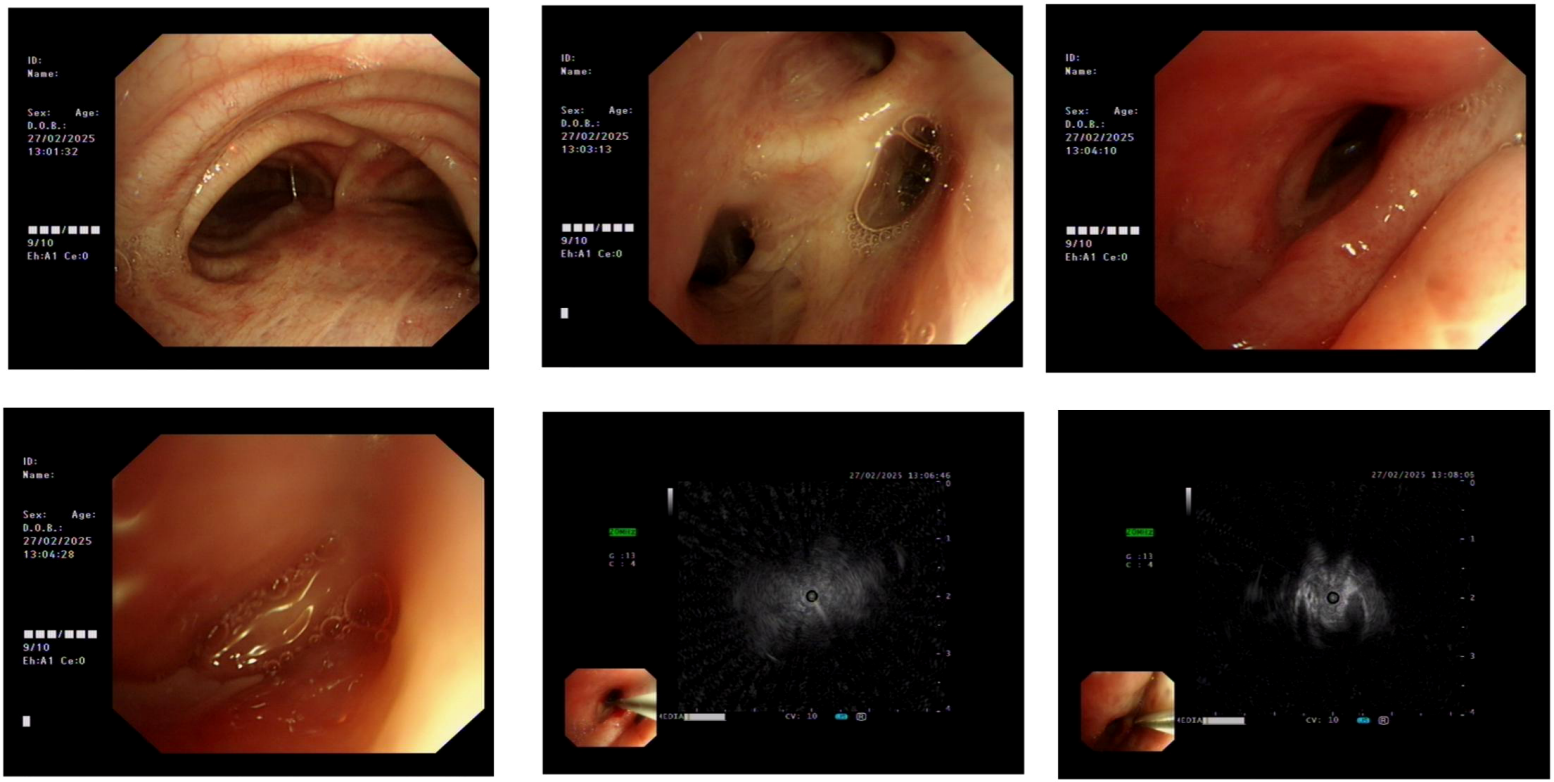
External compression-induced stenosis was observed at the bronchial openings of the right middle and lower lobes. Endobronchial ultrasound (EBUS) and brush biopsy were performed for further evaluation.
